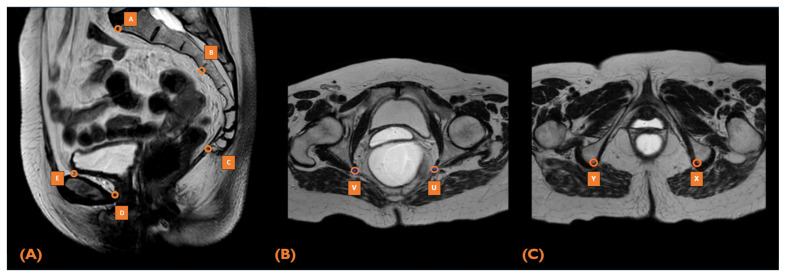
A visualization of MRI acquisition with the pelvimetry landmarks. (A) Sagittal MRI with the promontorium (A), S3-vertebrae (B), coccyx (C), dorsal part of os pubis (D), cranial part of os pubis (E). (B) Transversal MRI with the left and right ischial tuberosities (U and V). (C) Transversal MRI with the left and right ischial spines (X and Y). Abbreviations: MRI, magnetic resonance imaging.
